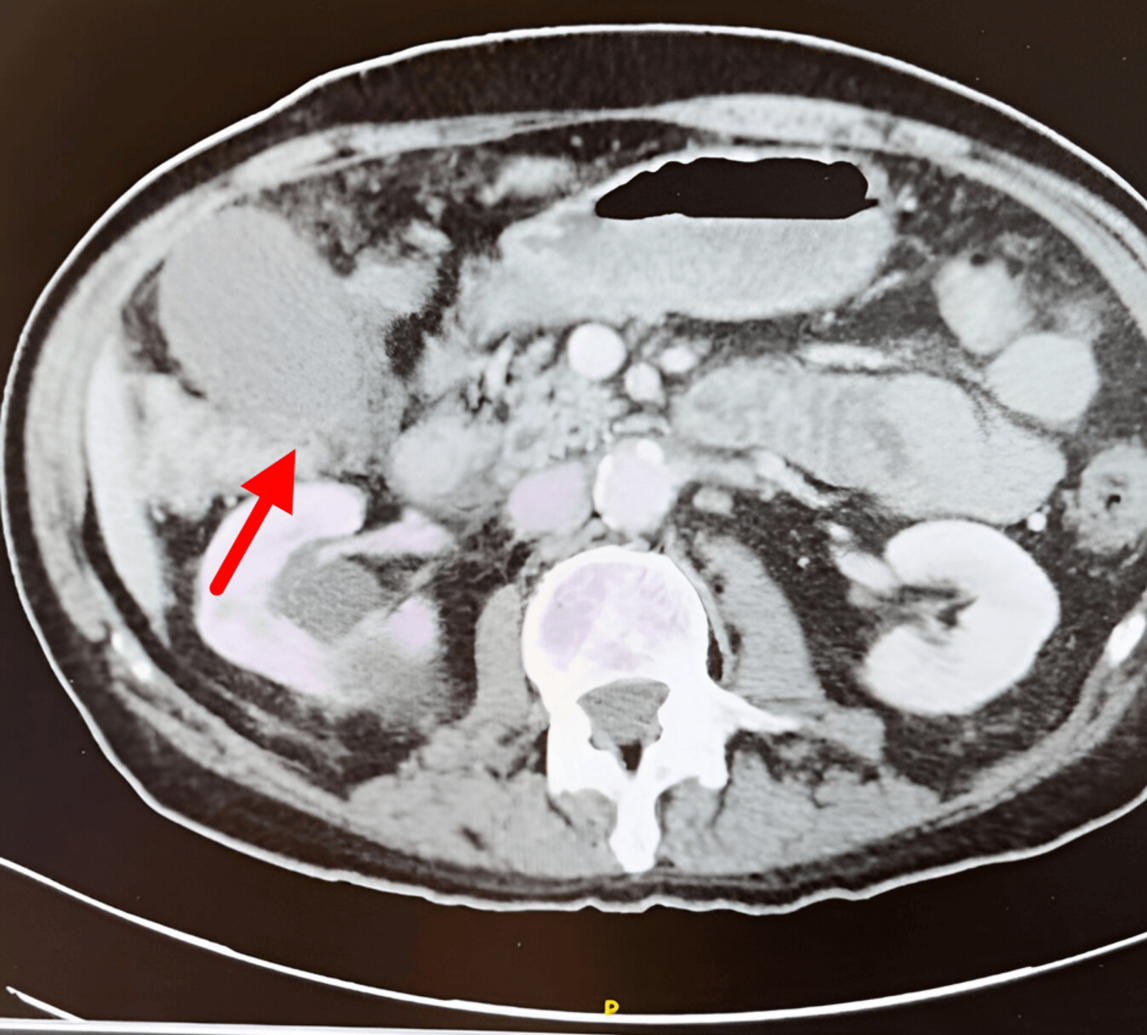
Coronal Slices of CT Thoracic Abdomen and Pelvis without contrast: Gallbladder Distension Arrow pointing at distended gallbladder with wall thickening.
